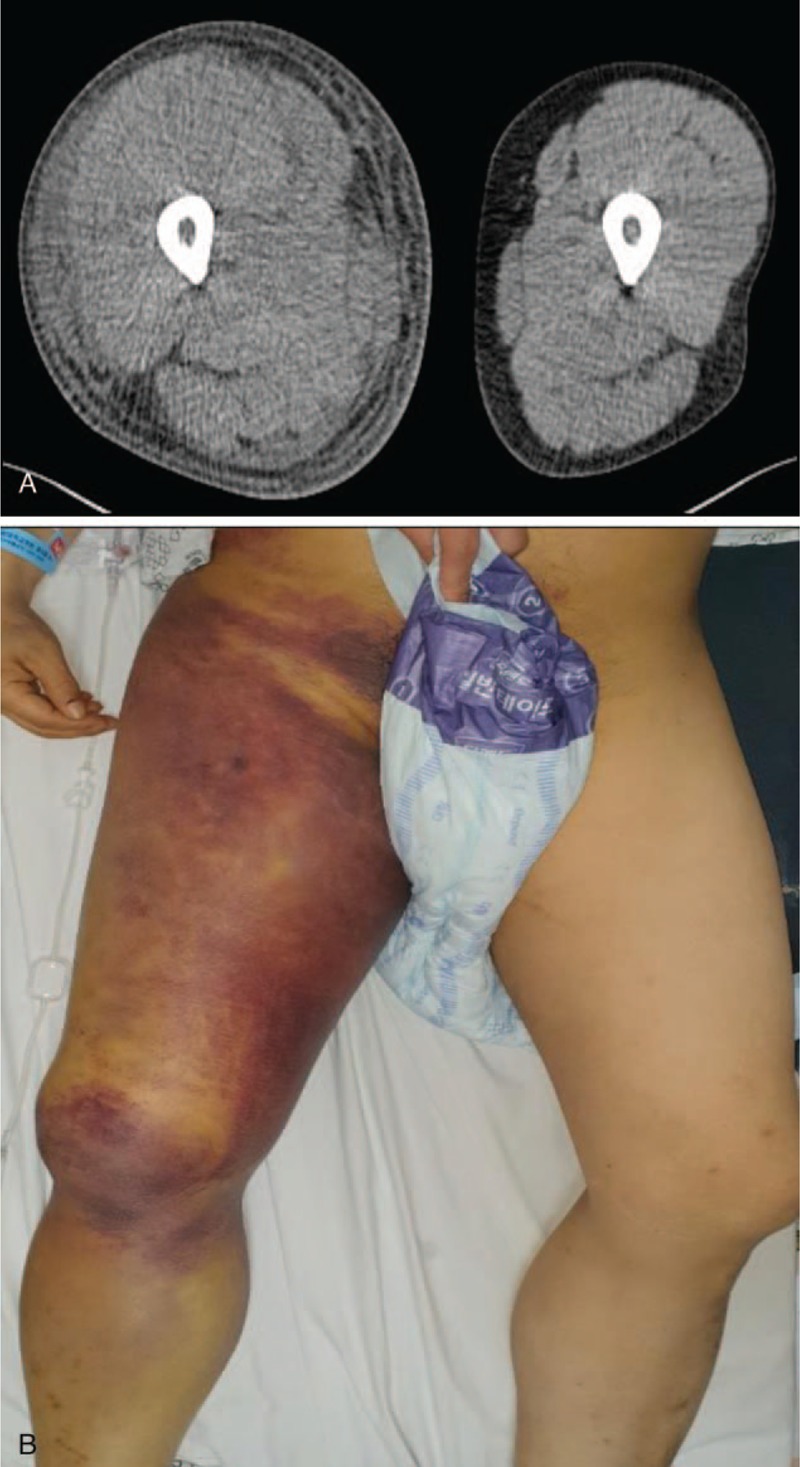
Computed tomography scan showing soft tissue swelling with some hematoma in right leg (A); and gross appearance of intramuscular hematoma of right thigh (B) on the day of admission.
